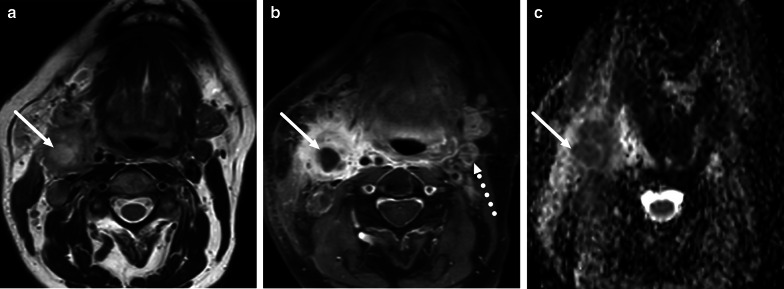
Infected necrotic lymph node metastasis (arrows) from oropharyngeal cancer misdiagnosed as suppurative lymphadenitis on axial T2-weighted (a) and fat-suppressed post-contrast T1-weighed images (b) and ADC (c). The patient had signs and symptoms of an acute neck infection. Low ADC suggests that the non-enhancing fluid collection is purulent. The cancer was not known at the time of imaging. Note the necrotic lymph node without signs of infection on the contralateral side (dotted arrow in b), suggestive of malignancy
